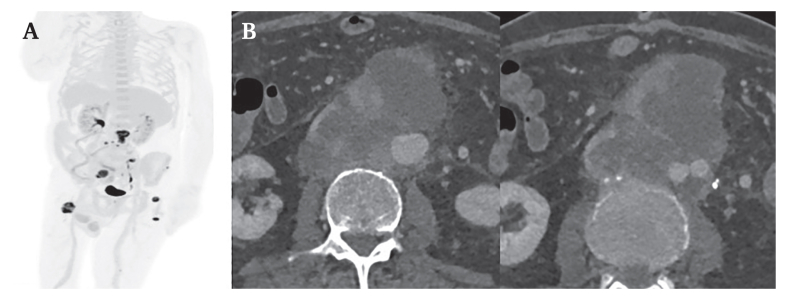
(A) Post-operative positron emission tomography computed tomography (CT) showing a highly metabolically active perivascular mass at the level of the infrarenal aorta with surrounding nodular lesions and bone metastasis. (B) Contrast enhanced CT three months post-operatively showing significant tumour progression at the level of the infrarenal aorta (left panel) as well as the aortic bifurcation.
